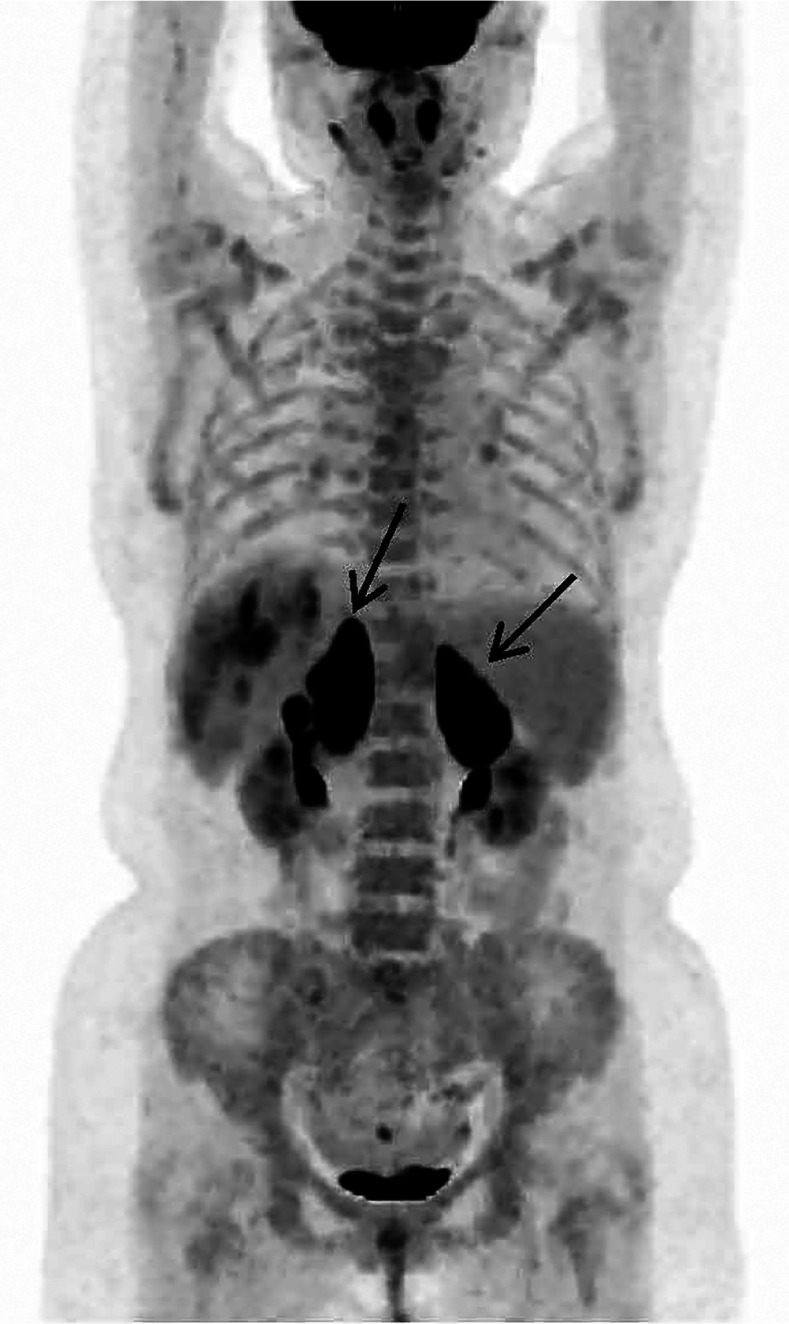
血管内大B细胞淋巴瘤患者2023年2月PET-CT检查结果（箭头所指为双侧肾上腺）
